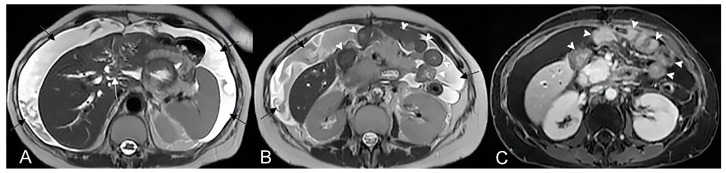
A case of radiation enteritis in a 54-year-old female patient with Klatskin tumor. (A) Axial T2-weighted image shows an intrahepatic Klatskin tumor (arrow), with dilatation of the intrahepatic bile ducts (asterisks). (B) Axial T2-weighted and (C) axial T1-weighted after gadolinium injection images on a different plane display diffuse thickening of upper small bowel loops (arrowheads), due to radiation therapy for Klatskin tumor. A massive amount of free abdominal fluid is also evident (black arrows in (A,B)).
